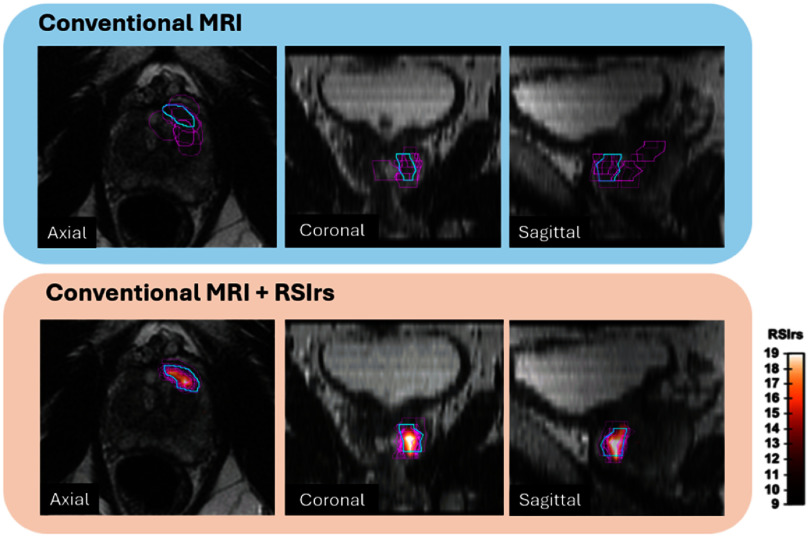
RSIrs maps improve the accuracy of participant target volumes. Expertly defined consensus volume is shown in cyan. Select participant volumes are highlighted in magenta. With RSIrs, participant volumes showed better agreement with the expert compared to conventional MRI alone.
